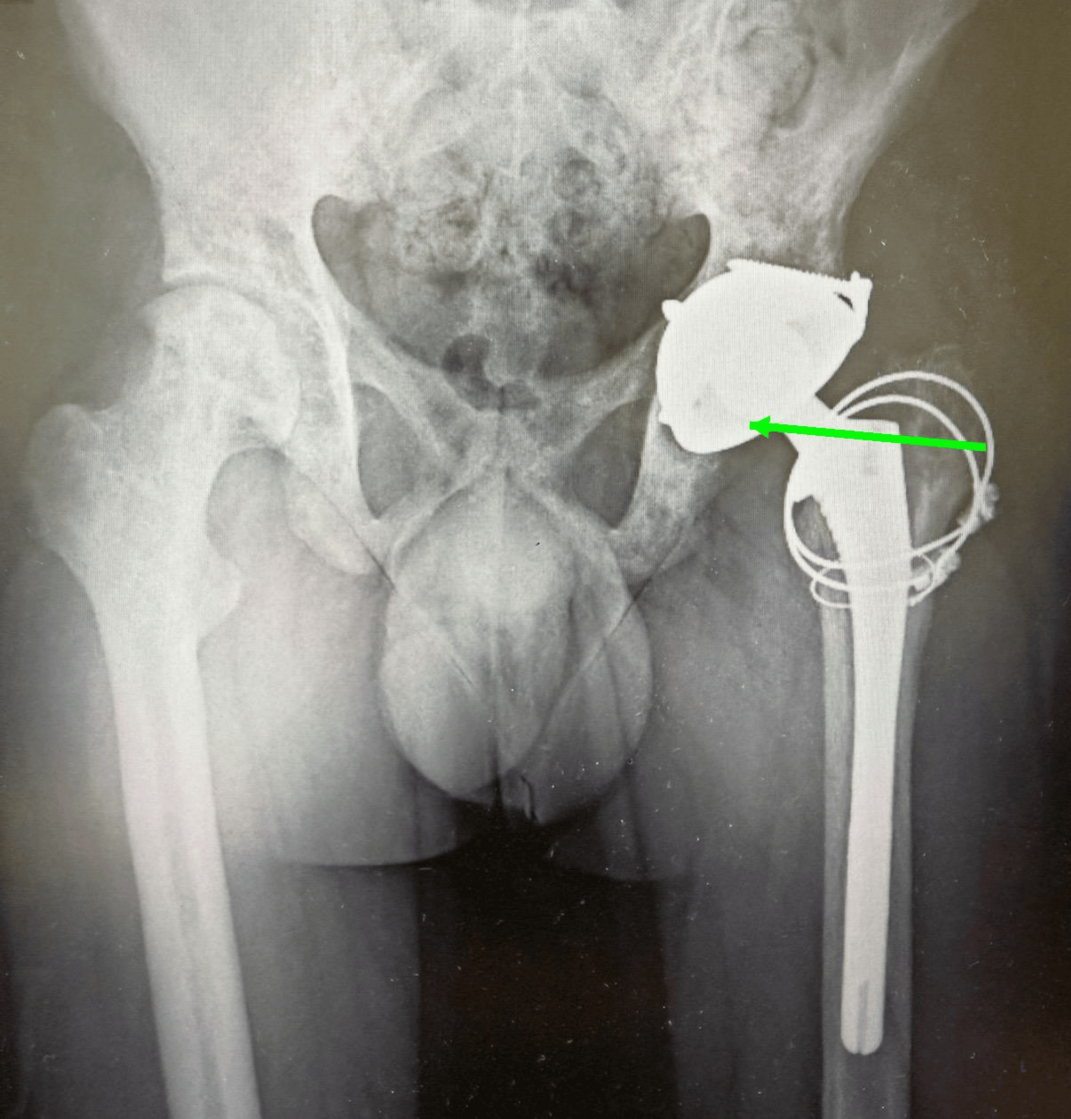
Immediate postoperative X-ray following second-stage THA Uncemented dual mobility implant following second-stage THA (green arrow). THA: total hip arthroplasty.
